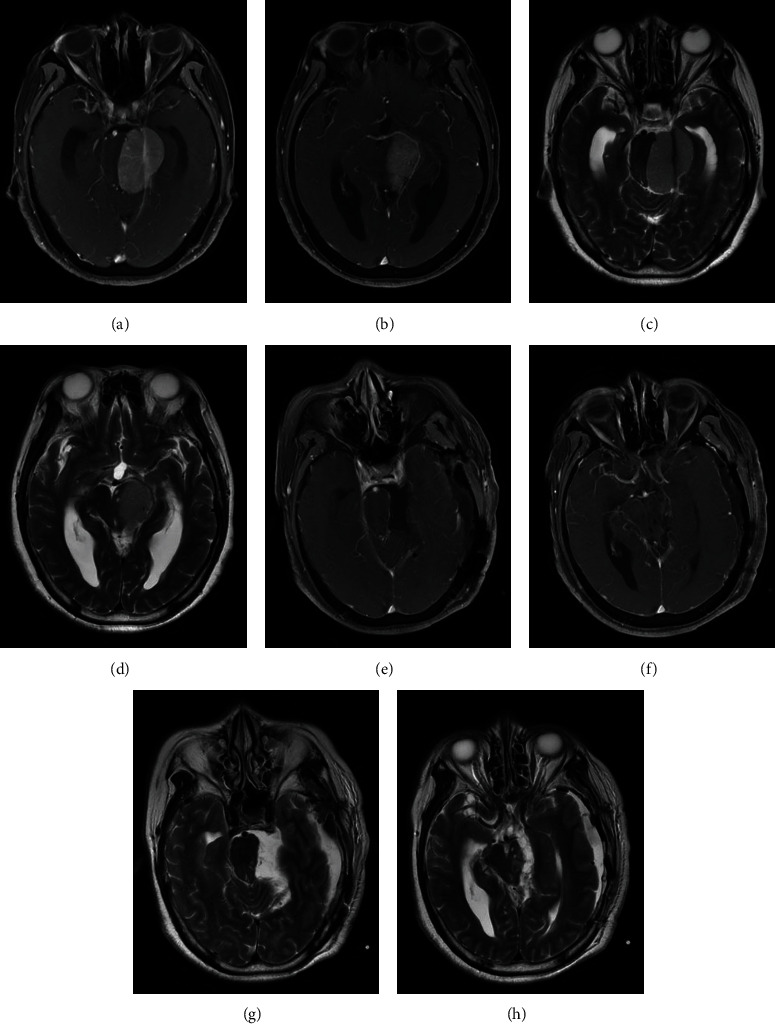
MRI obtained before and after surgical treatment with retractorless technics. (a, b) The preoperative enhanced MRI showed the tumor in the left petroclival region. The brainstem was squeezed to the right. (c, d) T2-weighted MRI showed no preoperative edema but two infarction lesions in the brain stem. (e, f) Postoperative enhanced MRI demonstrated a complete tumor resection. (g, h) Postoperative T2-weighted MRI showed no brain injury in the left temporal lobe.
